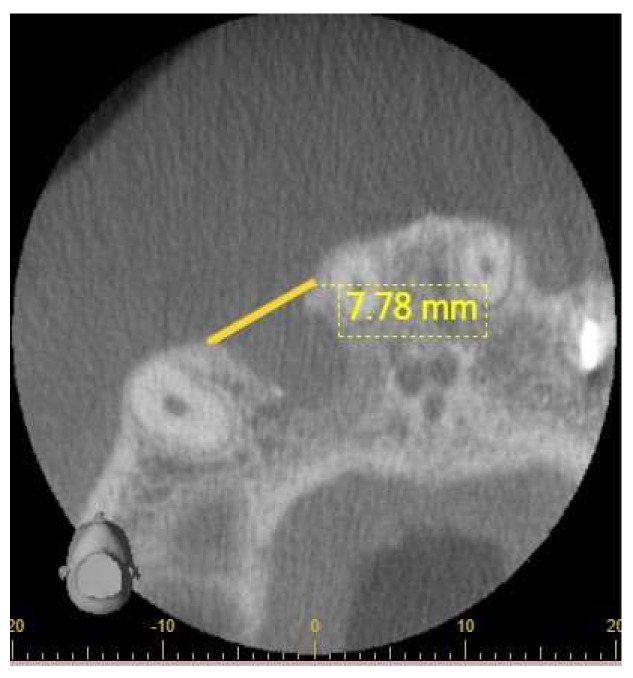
Periapical lesion measured on a CBCT image in mm in the MD direction.
